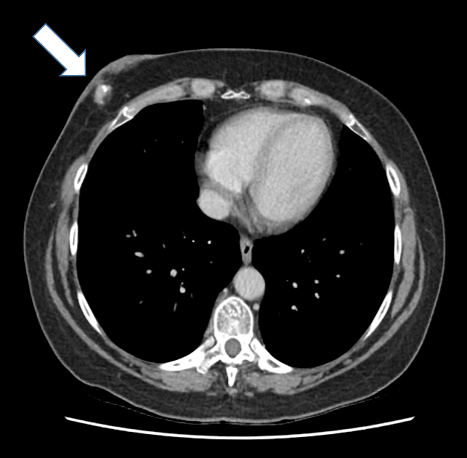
Axial CT slice of the patient’s chest showing the suspicious mass embedded in the right chest wall, at the position of the deep inferior epigastric perforator reconstruction. An area of calcification is visible within the lesion.
